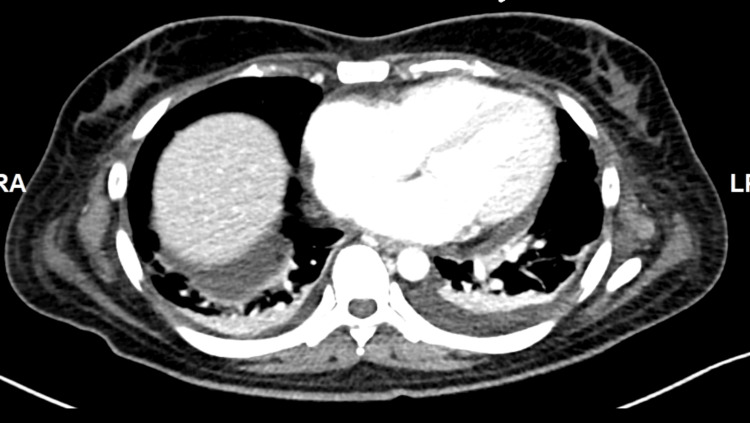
CT chest with contrast (axial) Axial contrast-enhanced computed tomography (CT) of the chest demonstrating bilateral pleural effusions, left greater than right, with increased density at the lung bases consistent with atelectasis; pneumonia cannot be excluded. CT: computed tomography
